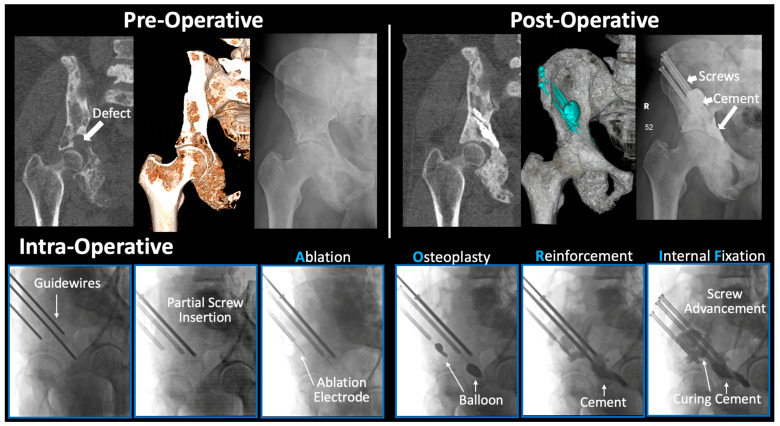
AORIF Procedure Overview. (Top Panel (from left to right)): Radiographical imaging of 62M with metastatic prostate cancer. One week pre-operative (from left to right) imaging demonstrating osteolytic acetabular defect with coronal CT, 3D rendering (internal view), and anterior-posterior pelvis X-ray. Post-operative imaging taken 12-months post-AORIF with PMMA cement and three cannulated screws. (Bottom Panel (Intra-Operative)): Initial guidewire placement with partial screw insertion for biopsy. Radiofrequency ablation initiated through insertion via cannulated screws. Balloon inflation creates space and boundaries to facilitate PMMA cement injection. Reinforcement with PMMA cement mixed with Zoledronate provides thermal necrosis of tumor cells and pain relief. Screws are then advanced into their final position in the curing cement for stabilization. AORIF: ablation, osteoplasty, reinforcement, and internal fixation. PMMA: polymethylmethacrylate.
